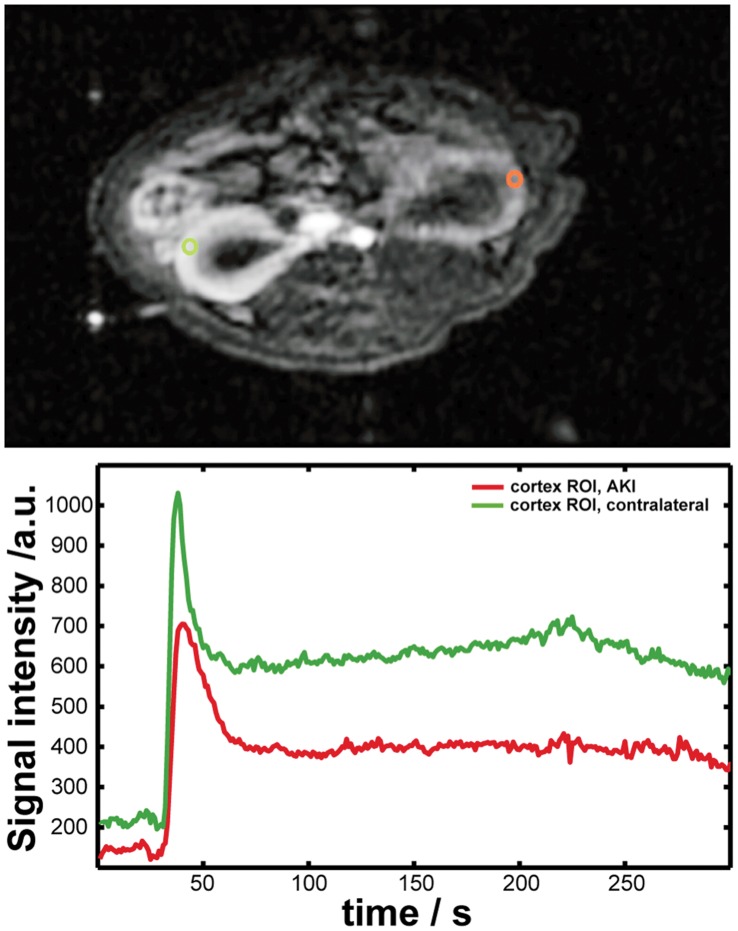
Time course of contrast uptake in DCE-MRI. The lower panel shows two exemplary DCE-MRI time courses of the tissue signal intensities (B), i.e. the contrast uptake in two cortical ROIs. In the upper panel (A) the position of the according ROIs is depicted. One ROI was placed in the healthy kidney (green), one in the diseased kidney (red). Please note that choice and shape of the ROIs in this figure are different from the ones drawn for the data evaluation.
